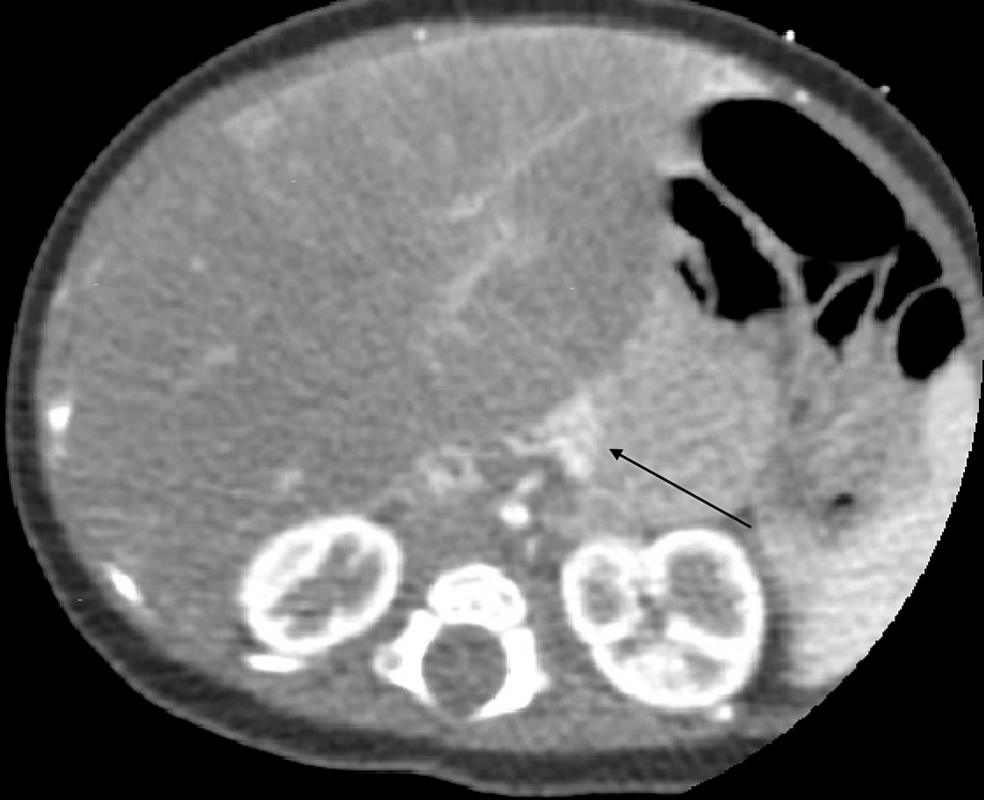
Axial CT view showing extralobar pulmonary sequestration (black arrow)
